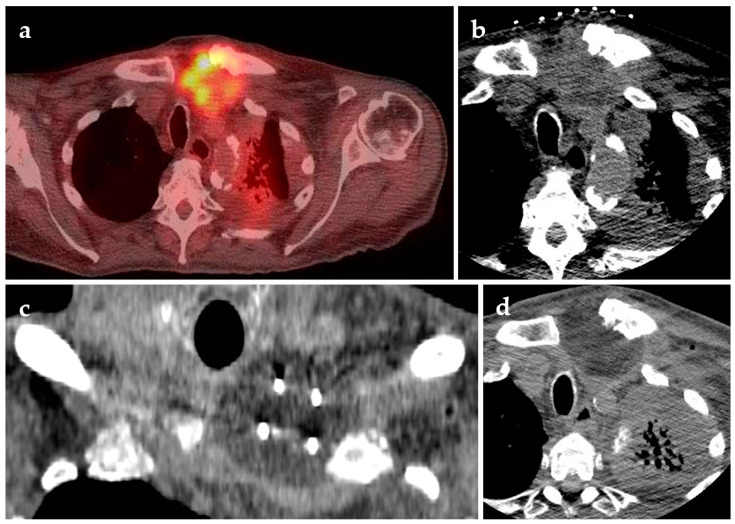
78-year-old female diagnosed with squamous cell carcinoma appearing as a left supraclavicular mass was treated with palliative ablation. The patient presented with left upper extremity pain, weakness and hoarseness of voice, as well as post radiation changes in the skin. (a) Supine axial FDG-PET scan demonstrating FGD-avid metastasis; (b) supine axial CT used for treatment planning; (c) supine coronal CT shows the probe positioning for cryoablation; (d) supine axial follow-up CT post-ablation.
